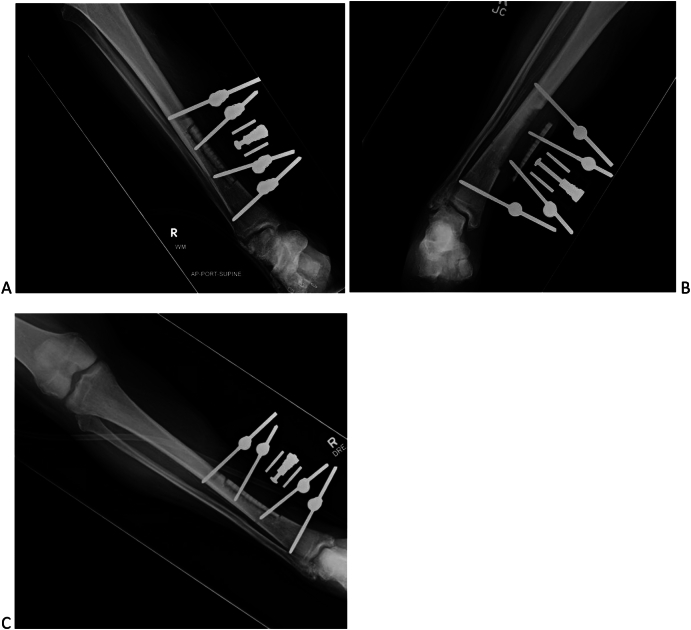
Radiographic dynamics of transverse tibial bone transport: A, latency period: B, distraction period; C, redocked transport segment.
